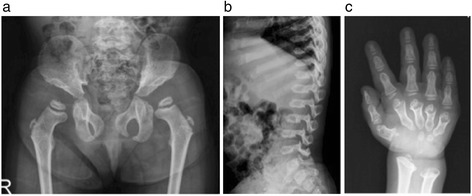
Radiographic evidence of Morquio A syndrome in a three-year-old female Morquio A patient (a-c). The pelvic radiograph (a) shows steep acetabulum with tapering ilium distally. Capital femoral epiphyseal dysplasia is noted. The lateral image of the thoracolumbar spine (b) shows uniform platyspondly with mild middle beaking. The image of the hand (c) shows strikingly short metacarpals with proximal coning and convergence toward centrally and phalanges that retain diaphyseal constriction. The distal radius and ulna inclined on their planes towards each other.
